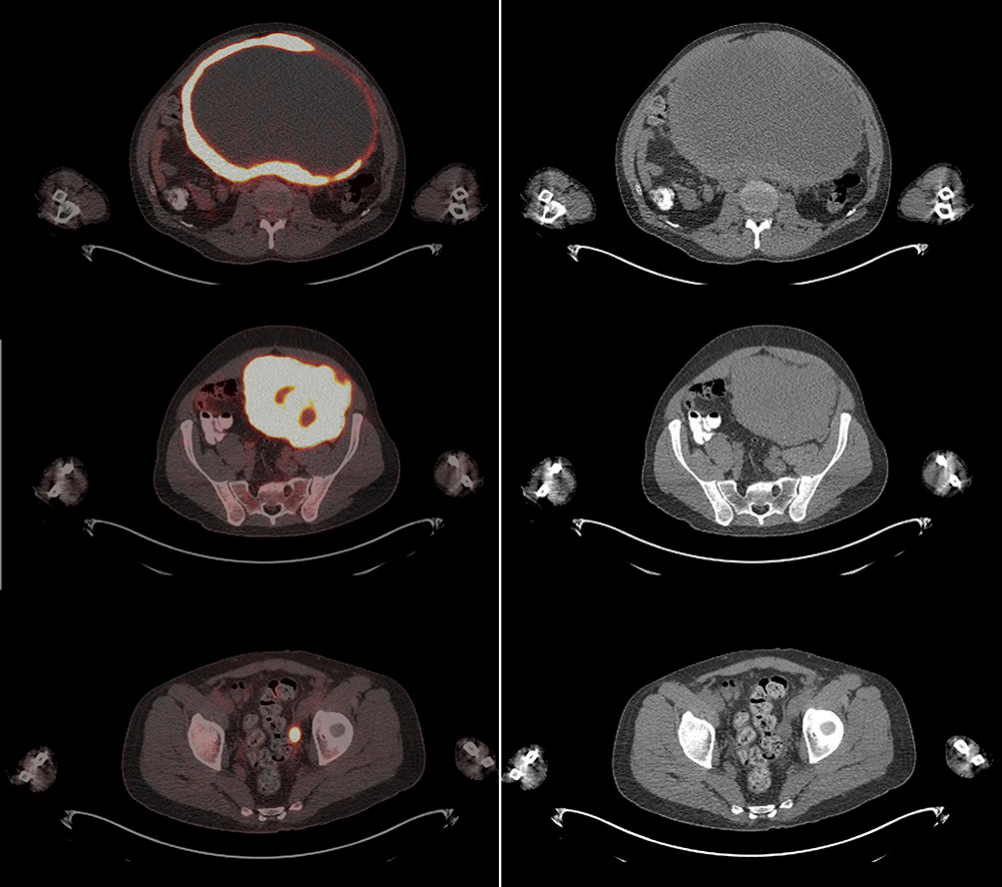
Staging positron emission tomography/CT fusion (left) and CT images (right) showing abdominal and peritoneal masses.
